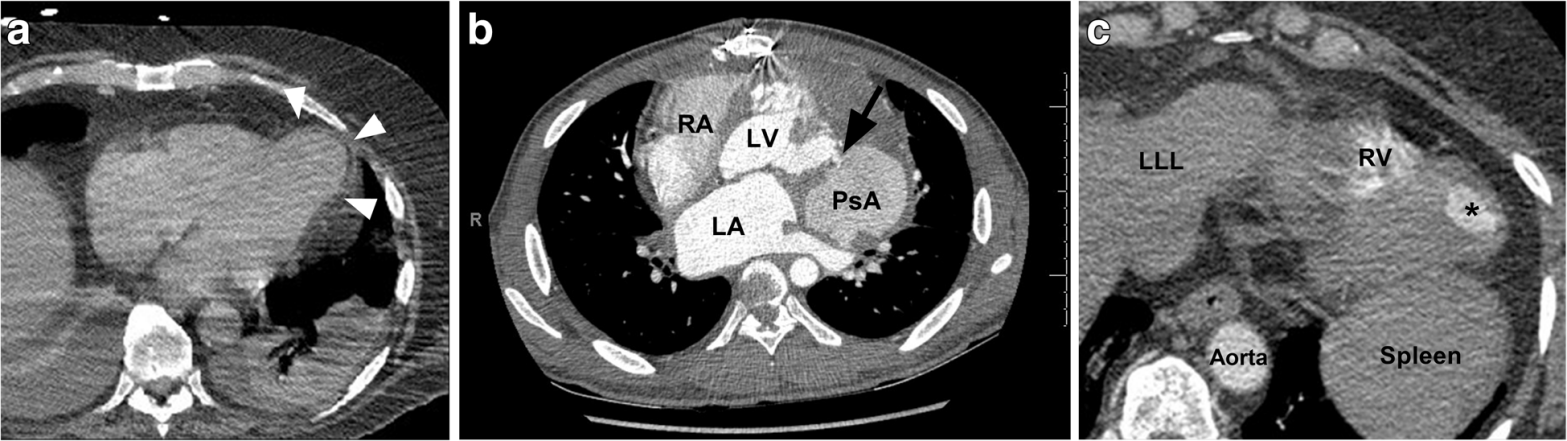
a Axial contrast-enhanced CT demonstrates a left ventricular true aneurysm (white arrowheads) in a 71-year-old male who was being evaluated for empyema. He had a history of myocardial infarction. b Axial CT image through the chest demonstrates a left ventricular pseudoaneurysm with a narrow neck (black arrow) in a young male being evaluated for fever and chest pain. *LA* left atrium, *RA* right atrium, *LV* left ventricle, *PsA* pseudoaneurysm. c Axial CT image through the lower chest and upper abdomen demonstrates a small apical left ventricular diverticulum (asterisk) in a 55-year-old male being evaluated for thoracic neoplasm. *LLL* left liver lobe, *RV* right ventricle
